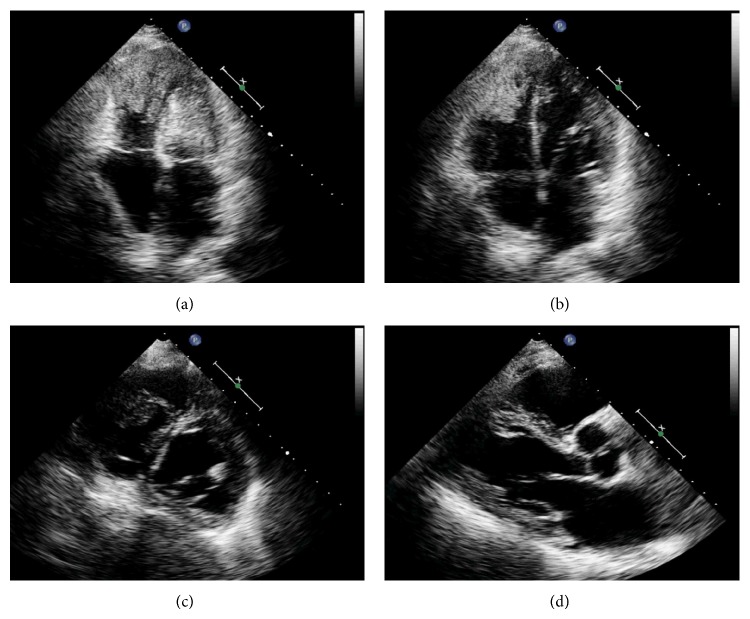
Transthoracic echocardiogram showing irregular mass in the right ventricle.
